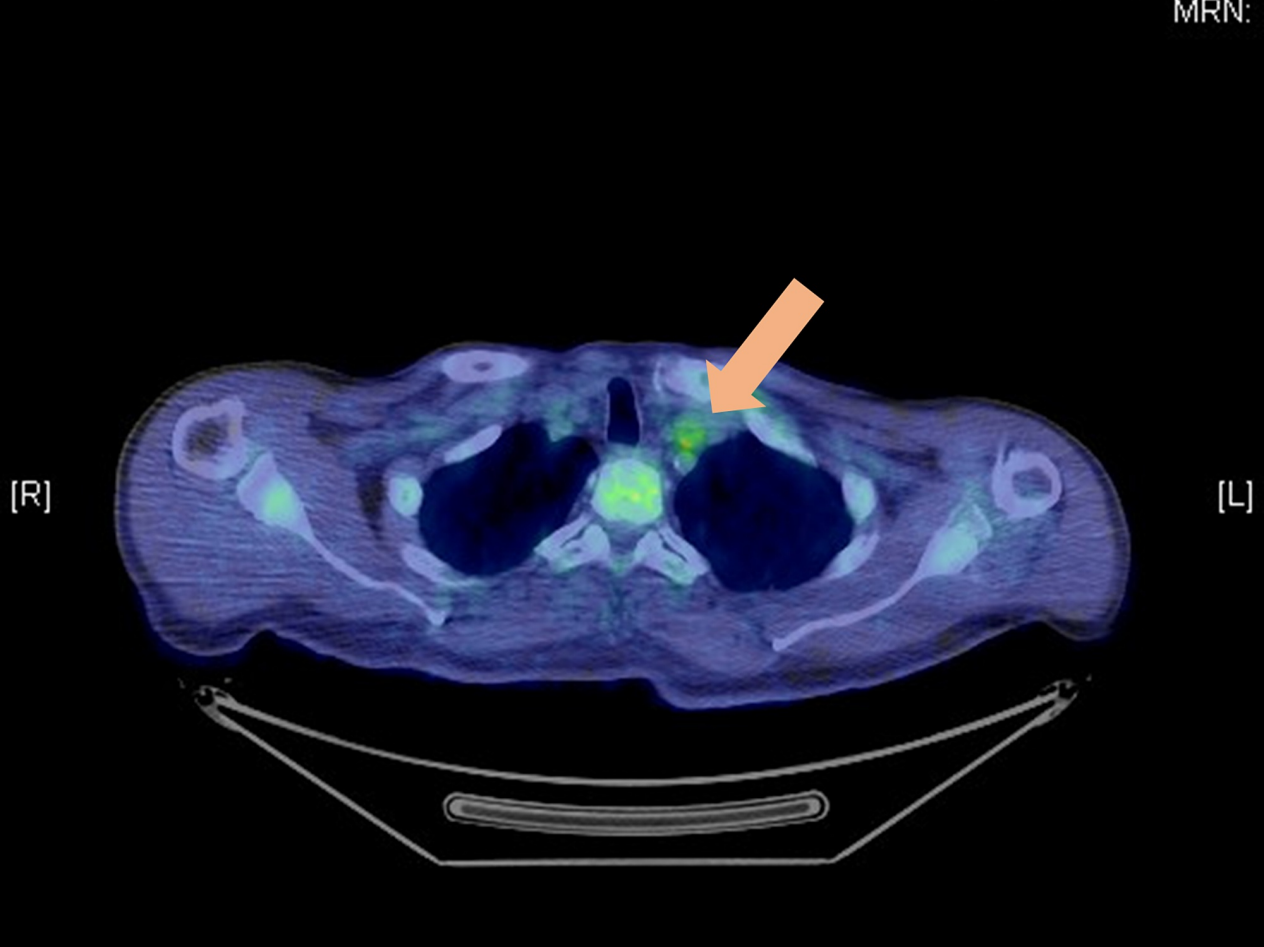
Positron emission tomography combined with computed tomography Positron emission tomography combined with computed tomography showing slightly higher fluorodeoxyglucose uptake in the sub/supra-clavicular lymph nodes
